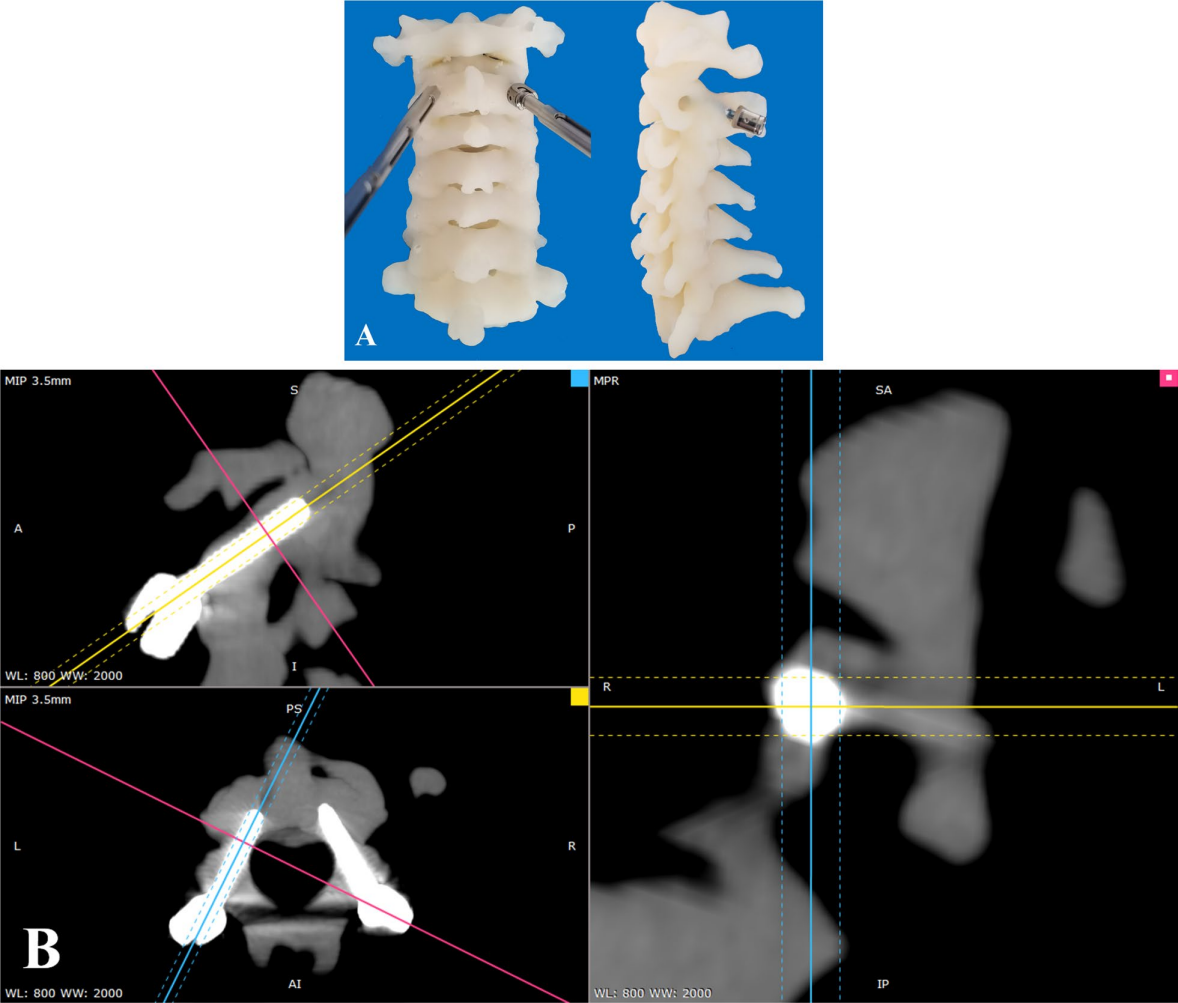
(A) C2 pedicle screws were inserted into 3D-printed bone model. (B) Postoperative CT multiplanar reconstruction was performed to assess the screw trajectory and cortical breach
